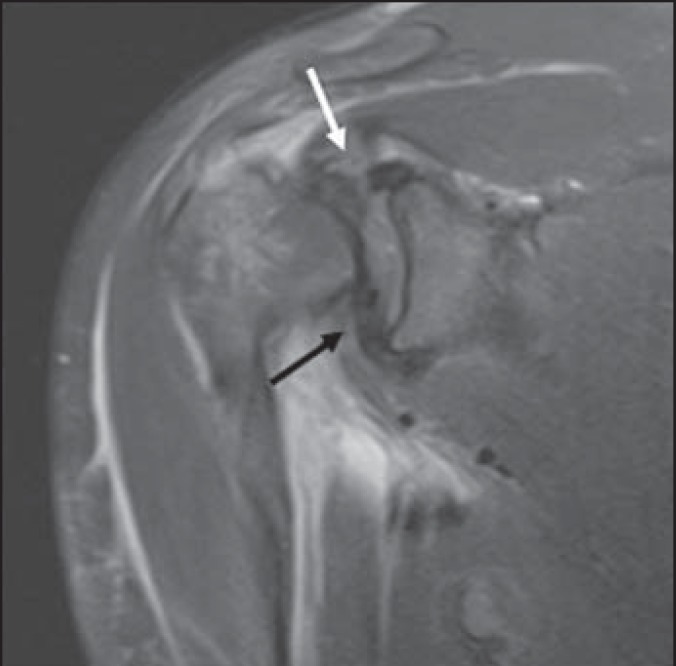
MRI, coronal section, intermediate weighting. The arrows identify rotator
